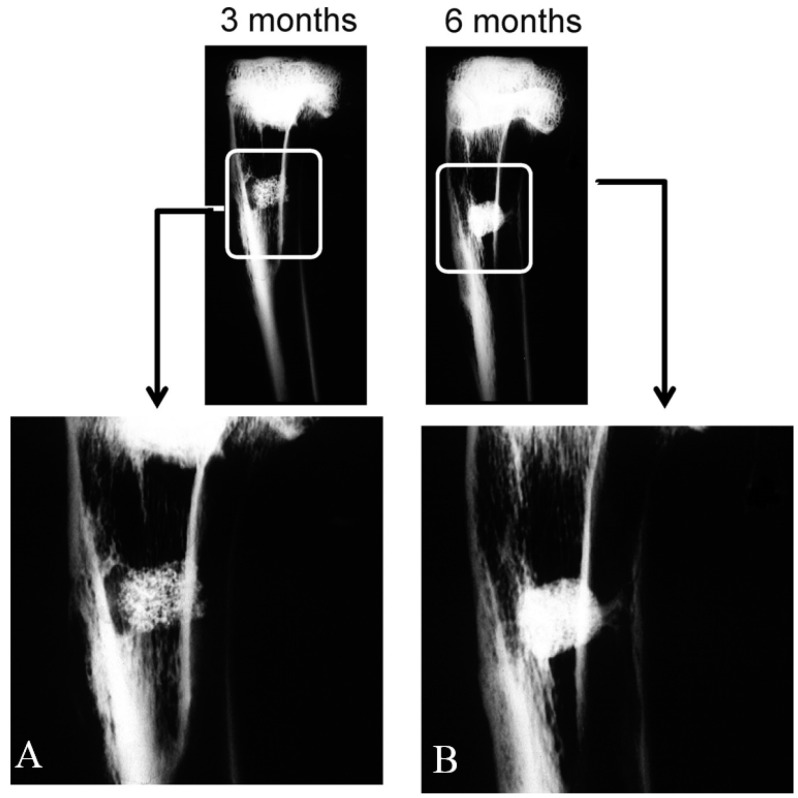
Antero-posterior X-rays of the bone section that contained the implants after three (A) and six months (B) post-implantation.
